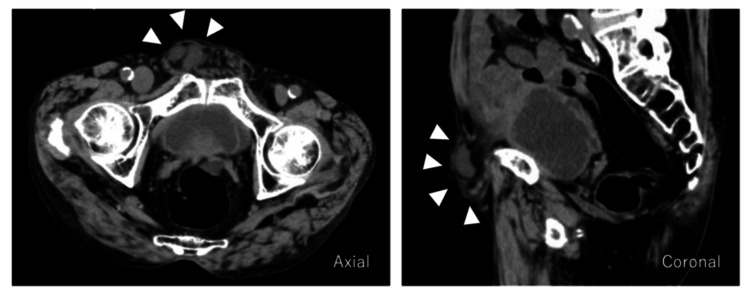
Preoperative plain abdominal CT (groin decompression prone positin) There was mild prolapse of fat tissue and a small amount of fluid in the right groin area (arrowhead) CT: computed tomography
